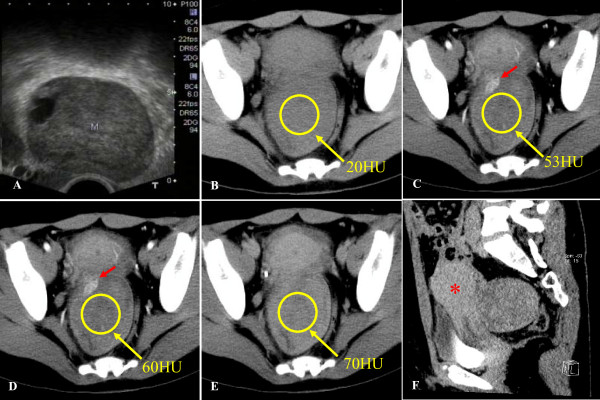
(A) Intra-vaginal ultrasonography of a pelvic mass. Intra-vaginal ultrasonography showed a 6.0 × 7.6 × 6.0 cm solid mass (M) between the uterus and the rectum. Dynamic contrast-enhanced computed tomography (CT) of the pelvis was used. (B) A non-enhanced CT scan showed the mass between the uterus and the rectum with nearly homogeneous density with a CT number of 20 Hounsfield units (HU). (C) Arterial phase, (D) venous phase, and (E) delayed phase contrast-enhanced CT showed that CT numbers of regions of interest within the mass (oval) gradually enhanced from 53 to 60 to 70 HU, respectively. A marked contrast-enhanced structure (red arrow) corresponding to tortuous vessels was shown with CT values of 140.0 HU in the arterial phase (C) and 111.6 HU in the venous phase (D). (F) Sagittal multi-planar reformation showed the mass (arrow) anteriorly growing and deforming the uterus (*).
